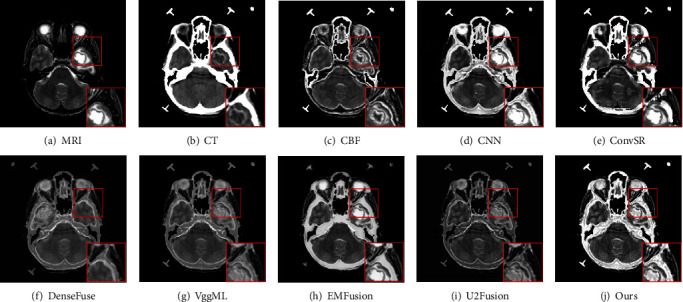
Visual comparison of our method with seven state-of-the-art methods on the second pair of MRI and CT images.
